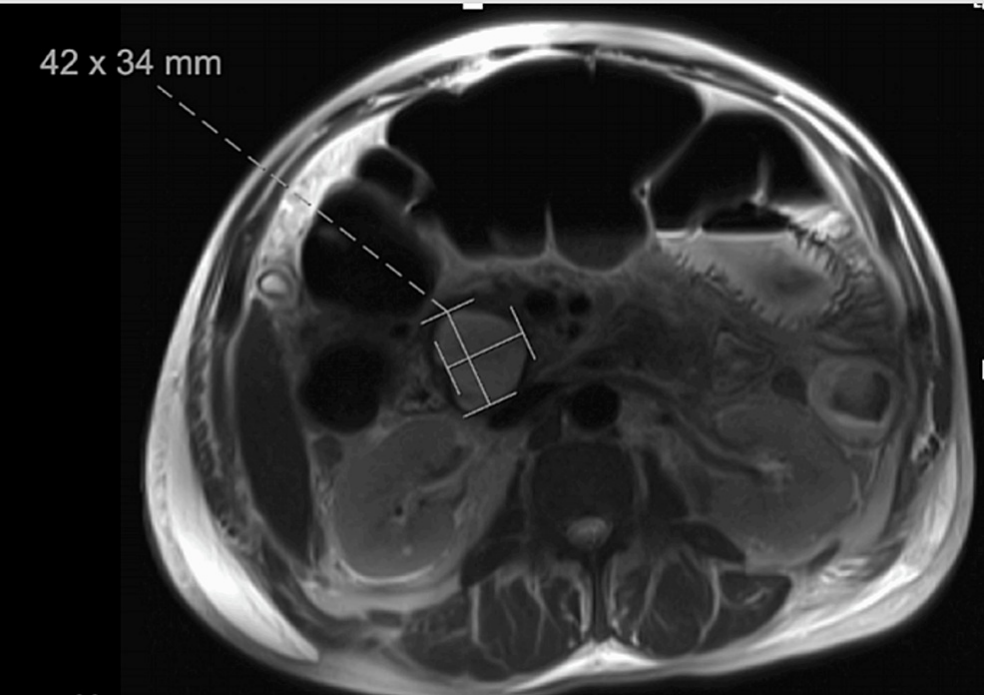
Case 2. An MRI revealing encapsulated complex fluid collection with rim enhancement measuring 4.2 x 3.4 cm, suggestive of a pancreatic pseudocyst without pancreatic ductal dilation or evidence of fistula.
